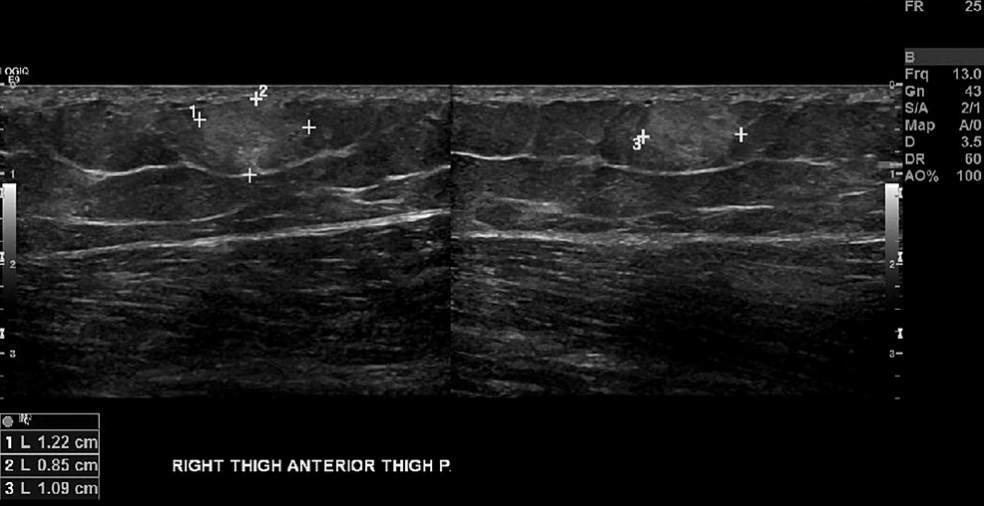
Ultrasound image of a 1.22 x 1.09 x 0.85 cm right anterior thigh soft tissue mass
